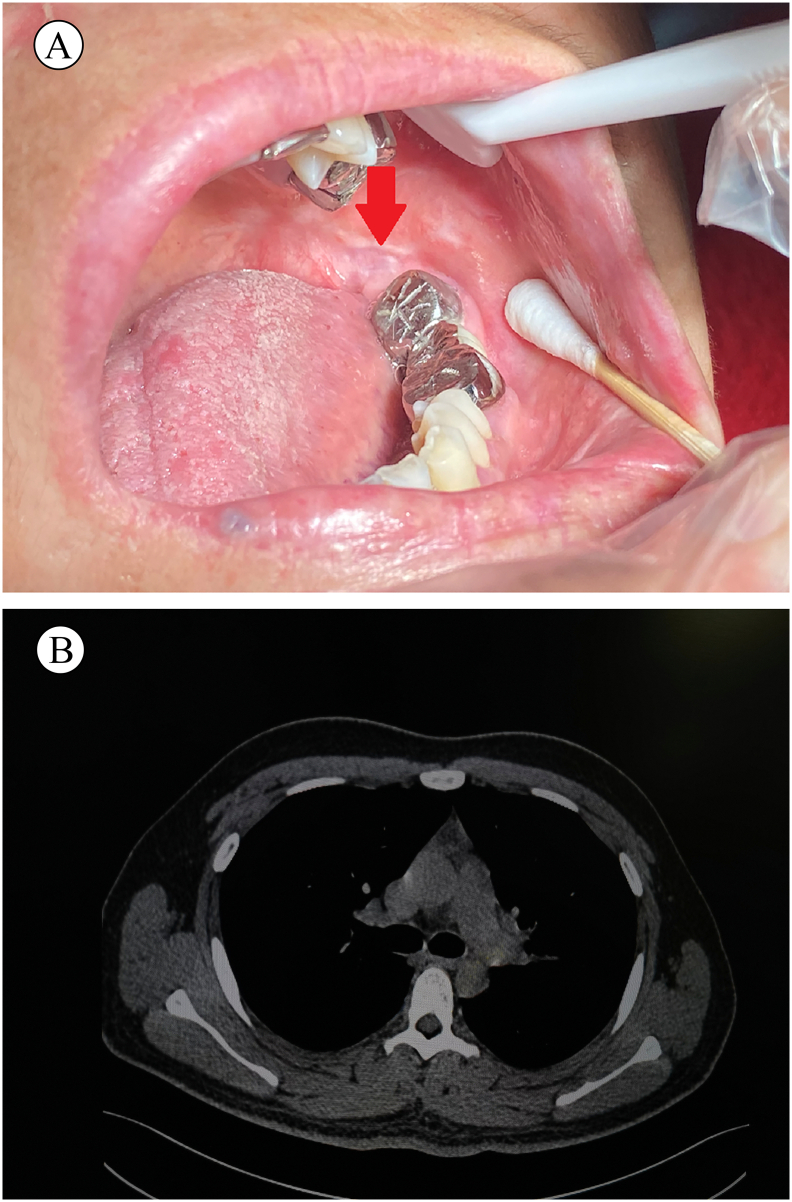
(A) Examination at the four-year follow-up reveals a smooth and intact mucosal surface (red arrow). A spiral CT scan of the lungs showed no abnormal metastatic lesions at two years (B). (For interpretation of the references to color in this figure legend, the reader is referred to the web version of this article.)
